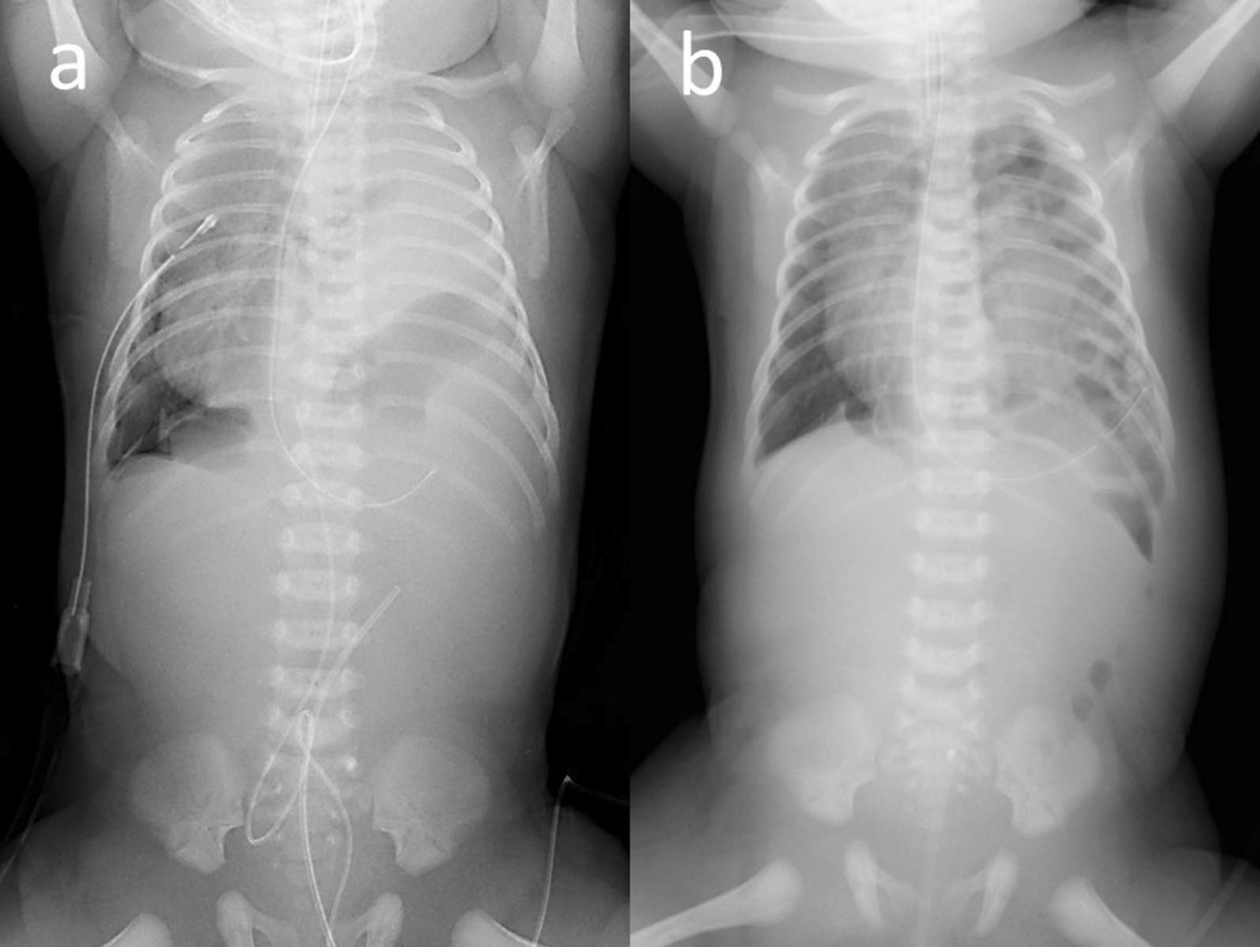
Abdominal and chest X-ray on admission (a) and day 4 (b). a Nitric oxide was inhaled and ventilator support was provided. A right pneumothorax was noted and required intrathoracic drainage until day 3. b The stomach, spleen, and small and large intestine were elevated in the thoracic cavity
